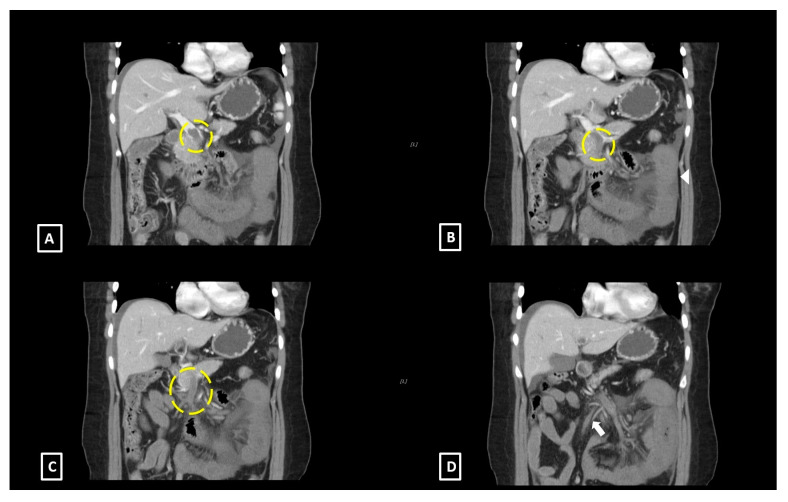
Coronal computed tomography images of the abdomen with intravenous contrast demonstrate: (A) A large filling defect within the main portal vein (yellow circle). (B) A large filling defect within the superior mesenteric vein (yellow circle) as well as circumferential thickening and hypoenhancement of the small bowel wall. (C) Extension of the thrombus to the superior mesenteric vein inferiorly and its branches (yellow circle). (D) Engorged superior mesenteric venous branches inferior to the thrombus (arrow).
